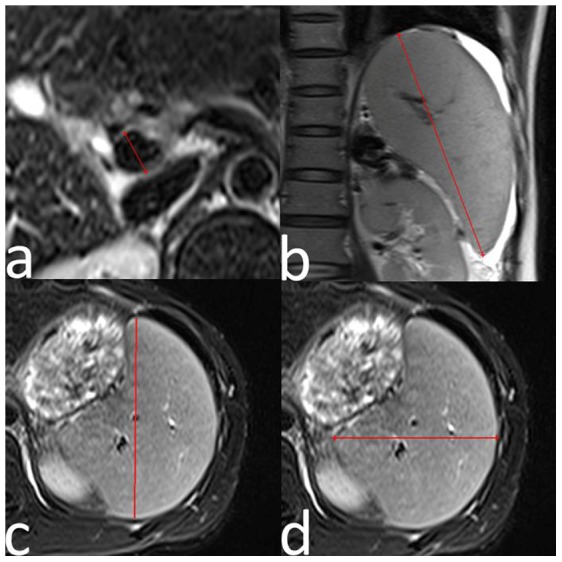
Measurements of portal vein diameter, SI, and platelet count/spleen diameter ratio. Measurement of portal vein diameter 3–4 slices above the junction of the superior mesenteric and splenic veins on transverse T2-weighted fat-suppressed images (a). Measurement of splenic craniocaudal (b), anteroposterior (c), and transverse (d) distance on coronal and axial T2-weighted images.
